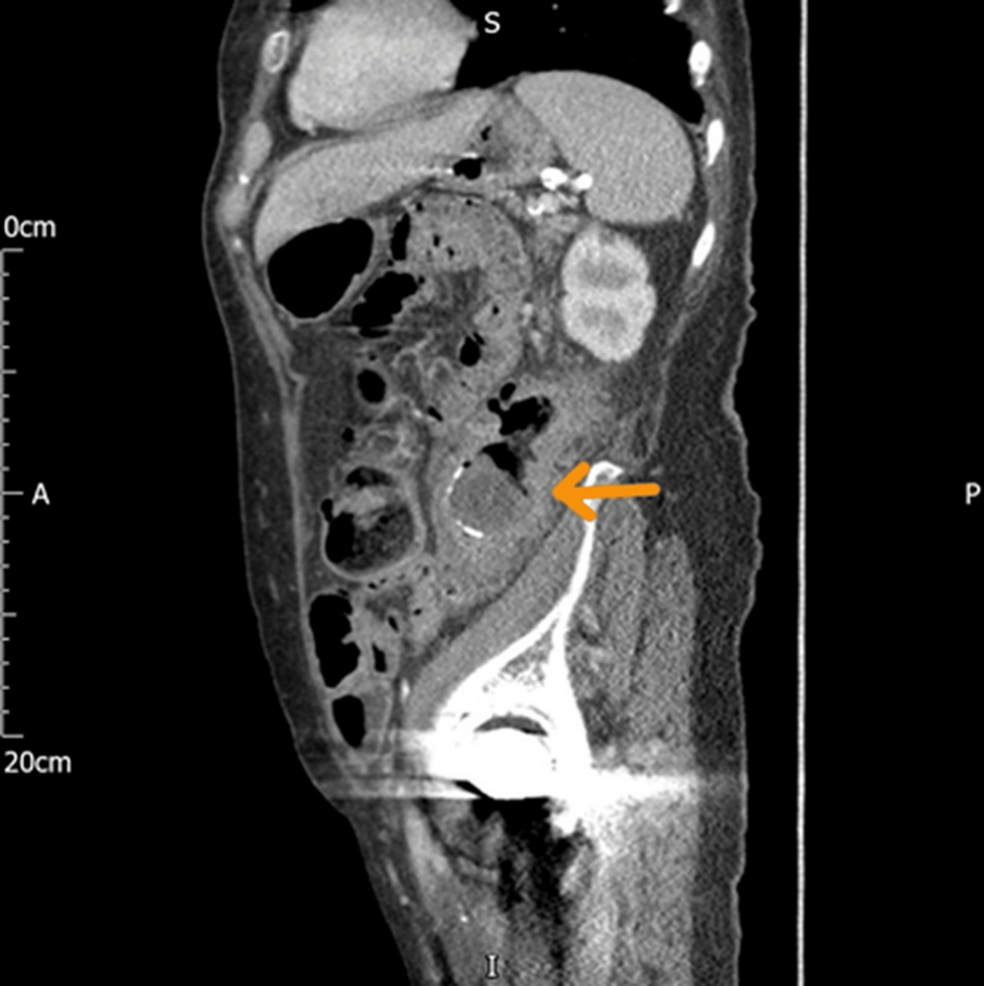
CT of the abdomen sagittal view shows gallstone in the colon (the brown arrow) CT: computed tomography
